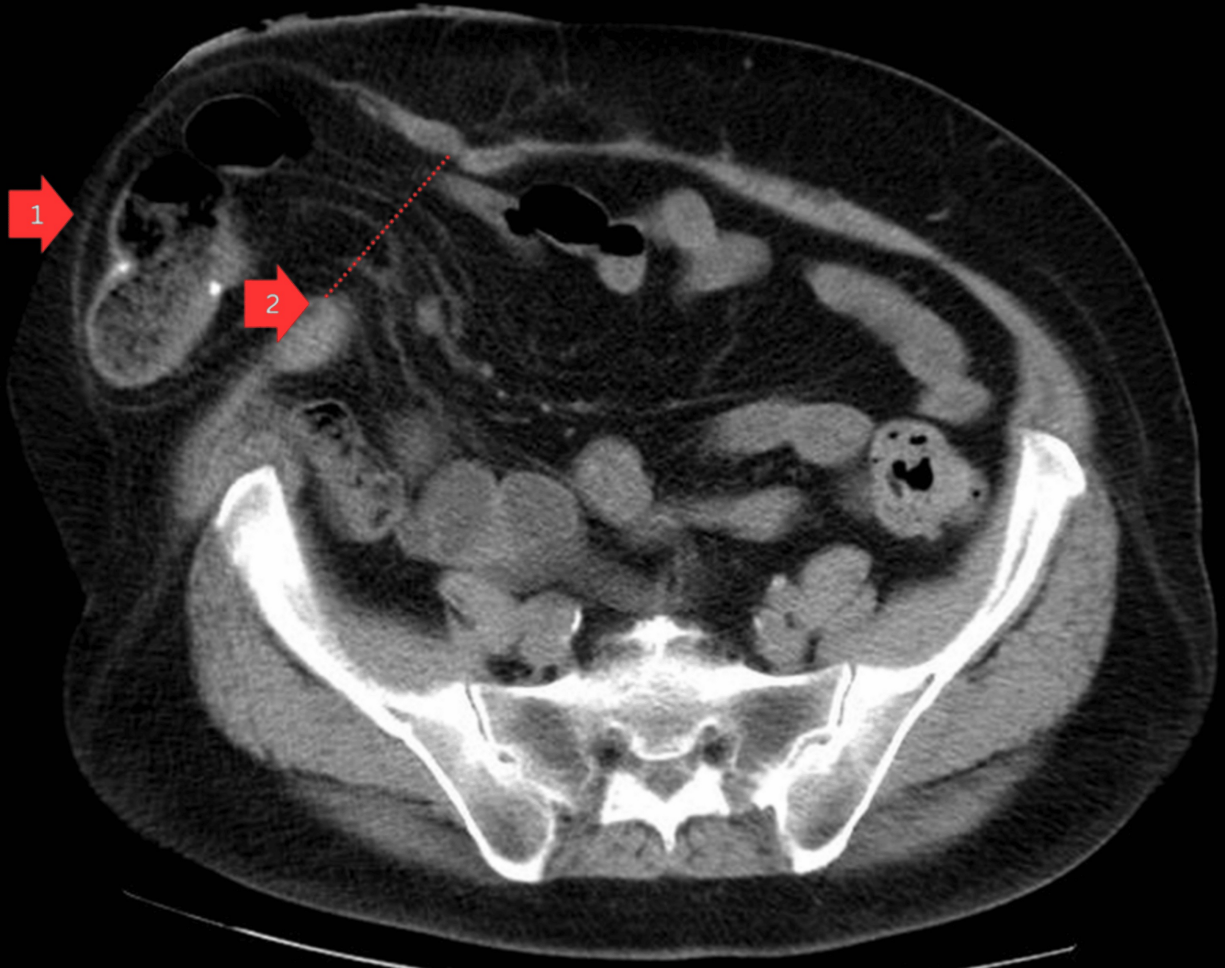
Abdominopelvic computed tomography axial imaging identifying parastomal hernia Arrow 1: hernial sac, Arrow 2: hernial orifice
